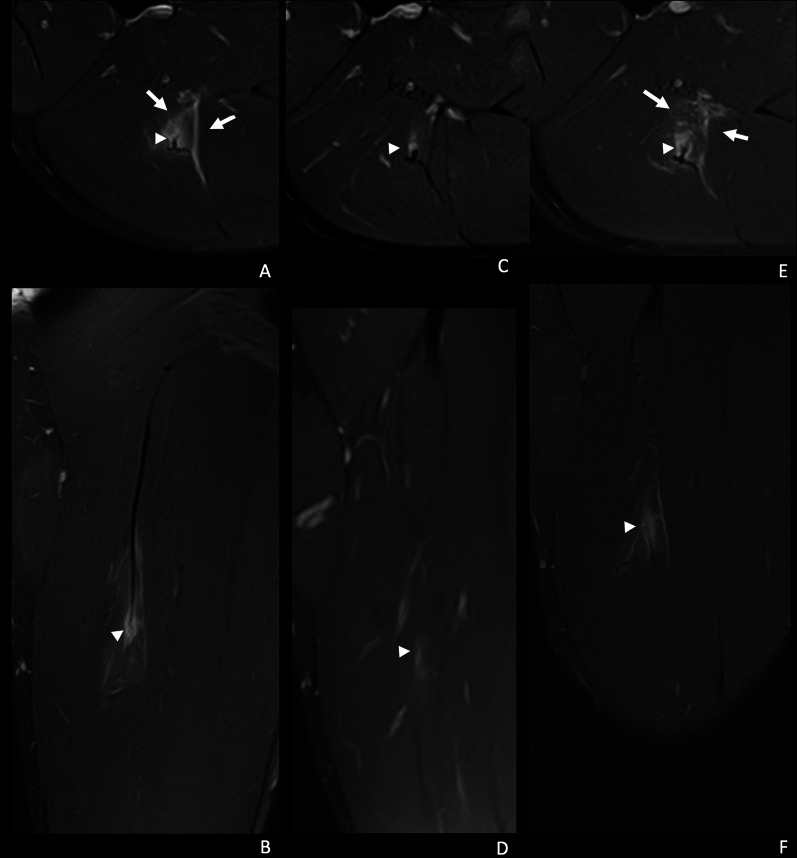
A 21-year-old right leg dominant football player. He presented during a training session, with tenderness in the middle third of the posterior aspect of the left thigh, and inability to continue training. A, B Axial and coronal T2-weighted fat-saturated images from a baseline MRI show myotendinous injury of the intramuscular portion of the proximal MTJ of the biceps femoris long head, I Mp 3r 0 (MLG-R classification [33]). There is a transversal tear of the connective tissue with loss of tension (arrowhead) and interstitial and intermuscular oedema (arrows). C, D Axial and coronal T2-weighted fat-saturated images of the pre-RTP MR (37 days post-injury), show a small transversal peripheral tendinous tear (arrowhead) with a trace interstitial oedema. E, F Reinjury 1 day later. Axial and coronal T2-weighted fat-saturated images show a thicker transversal tendinous tear with fibre muscle tear (arrowhead) and more interstitial oedema and intermuscular oedema (arrows)
